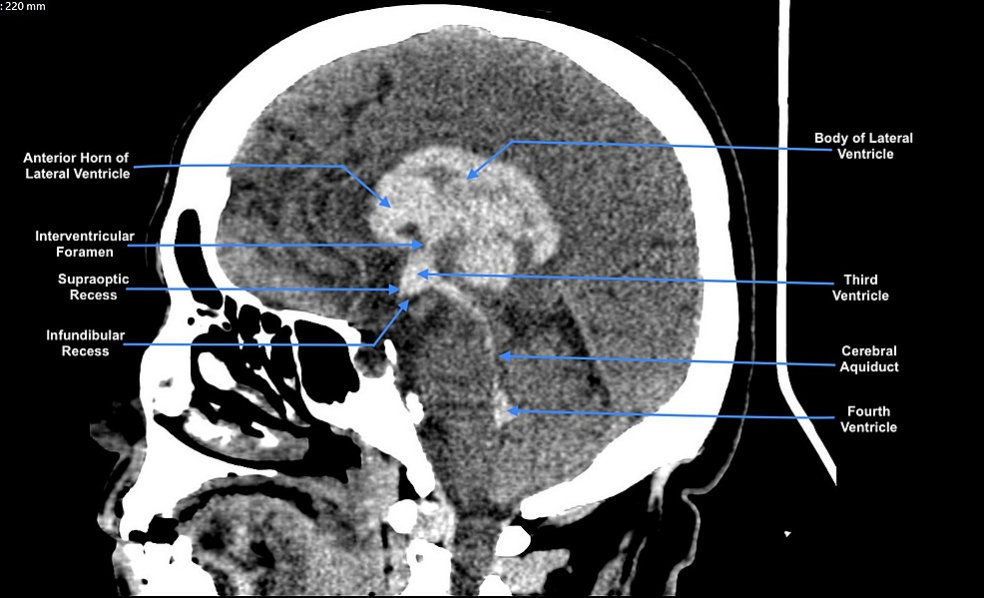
Non-contrast CT image of the brain, sagittal view. The labeled hyperdense area is a right-sided intraparenchymal hematoma casting the ventricular system and outlining the details of its anatomy [12].
